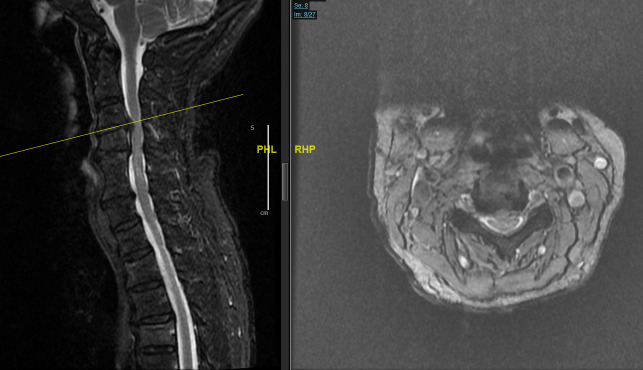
MRI T2-weighted sequences showing multilevel degenerative pathology most significant at C3–C4, where there is severe spinal canal stenosis with cord signal change suggesting myelomalacia.
